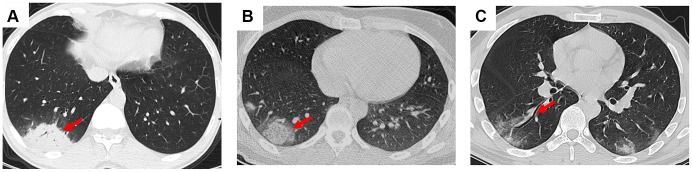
Consolidation, halo sign, and bronchiectasia in the CT scans of COVID-19 pneumonia patients. (A) Consolidation accompanying air bronchogram sign was found in the right lower lobe of a 46-year-old male patient; (B) Halo sign was observed in the right lower lobe of a 18-year-old male patient; (C) Bronchiectasia was observed in the right lower lobe of a 30-year-old male patient with bilateral ground-glass opacity. Typical lesions were marked with red arrows.
